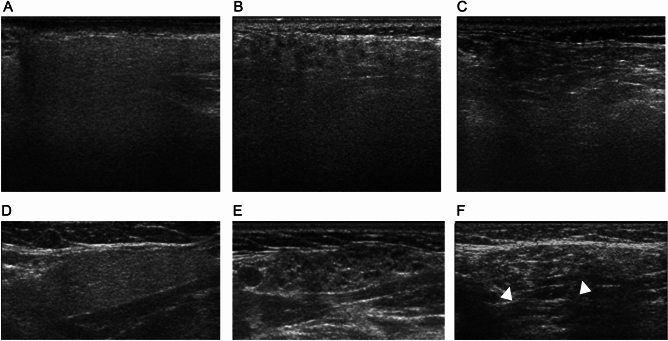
Representative ultrasonography findings of parotid and submandibular glands in patients with Sjögren's syndrome and those without Sjögren's syndrome. (A and D) Patients without Sjögren's syndrome. Ultrasonography shows hypoechoic areas (–) and hyperechoic bands (–) in parotid (A) and submandibular (D) glands. (B,C,E,F) Patients with Sjögren's syndrome. Hypoechoic areas (+) in parotid (B) and submandibular (E) glands. Hyperechoic bands (+) in parotid (C). Hyperechoic bands (+) and irregular margins (+) (arrowheads) in submandibular (F) glands.
